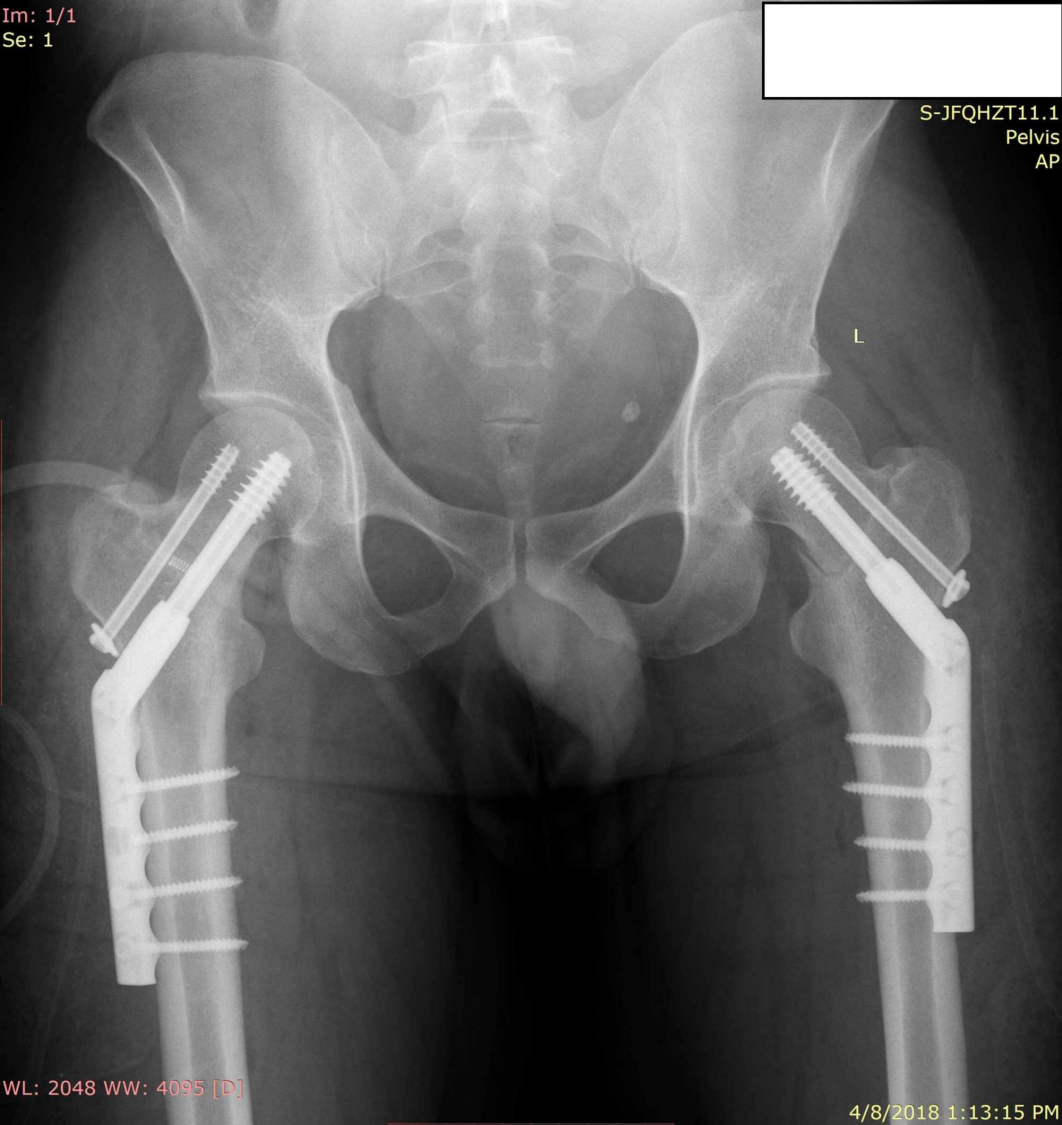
Immediate post-operative x-ray showing internal fixation using dynamic hip screw on both sides.
